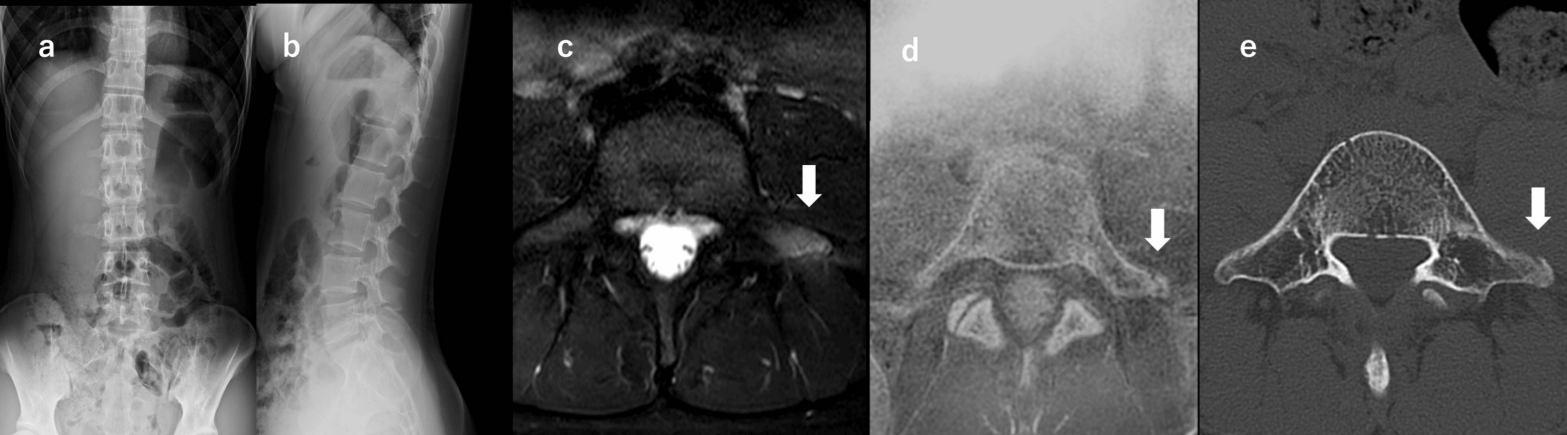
Sequential imaging findings of an L5 transverse process stress fracture in Case 2 (a, b) Plain radiographs showing no abnormalities, (c) Axial Short Tau Inversion Recovery (STIR) MRI demonstrating high signal intensity at the tip of the left L5 transverse process and adjacent soft tissue (white arrow), (d) MR bone imaging revealing a fracture line and avulsion at the tip of the left L5 transverse process (white arrow), (e) Axial CT image at four weeks confirming bone union (white arrow).
